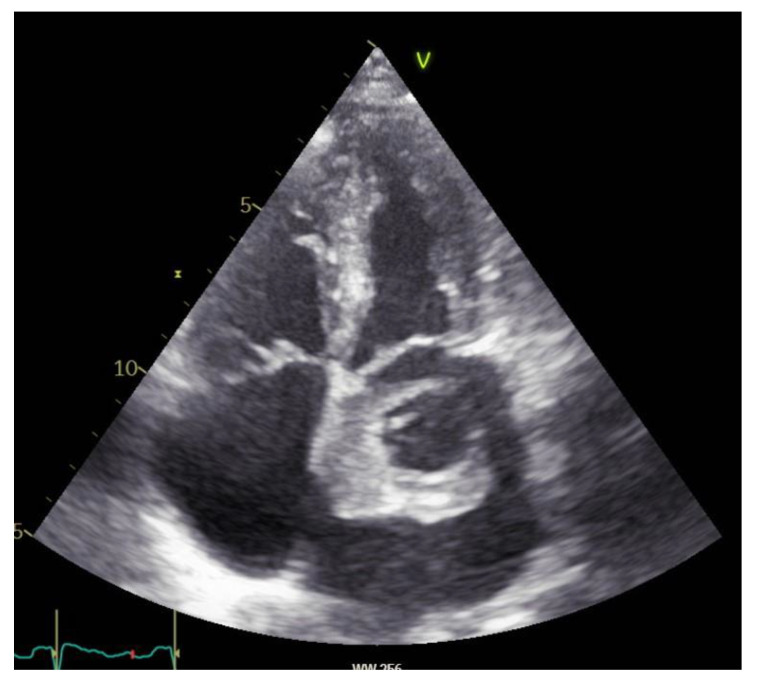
Echocardiographic imaging of a CM located in the left atrium.
